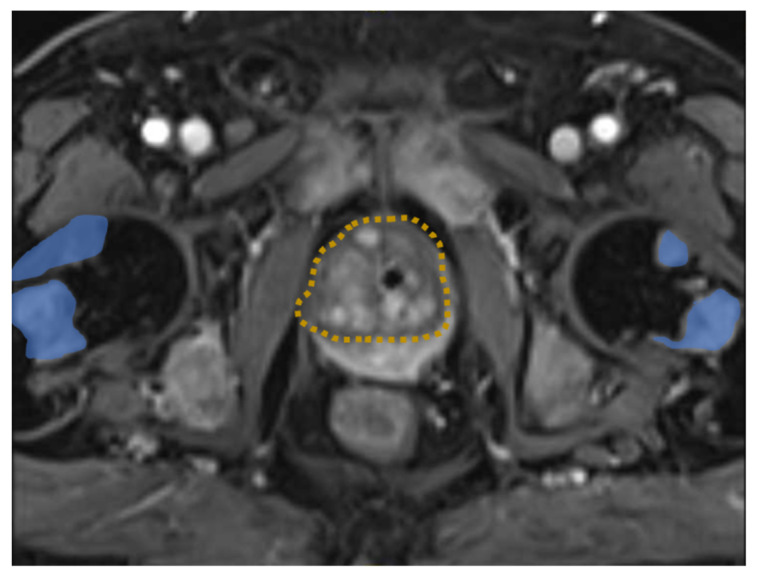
MRI image of the pelvis demonstrating prostate cancer (dashed orange) with multiple bone metastasis (blue).
